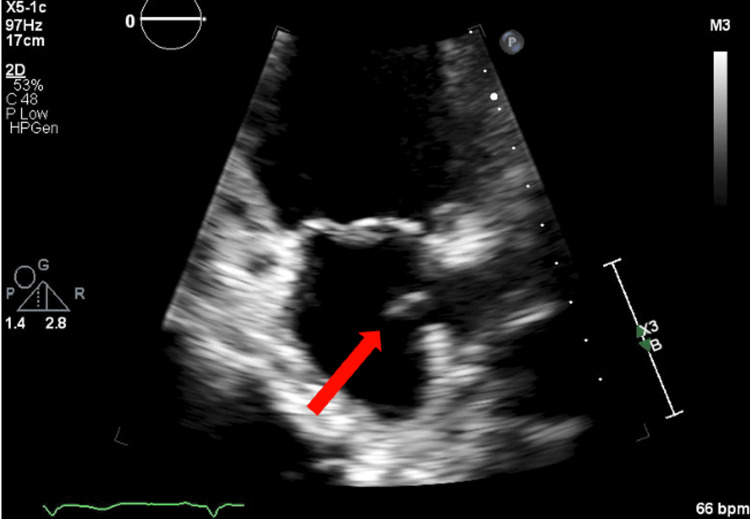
TTE in the apical right ventricle focused view, zoomed in on the right atrium showing a mobile echo-bright mass in the right atrium abutting the atrial septum with newly reduced EF of 40% and reduced right ventricular systolic function. TTE: transthoracic echocardiography; EF: ejection fraction.
